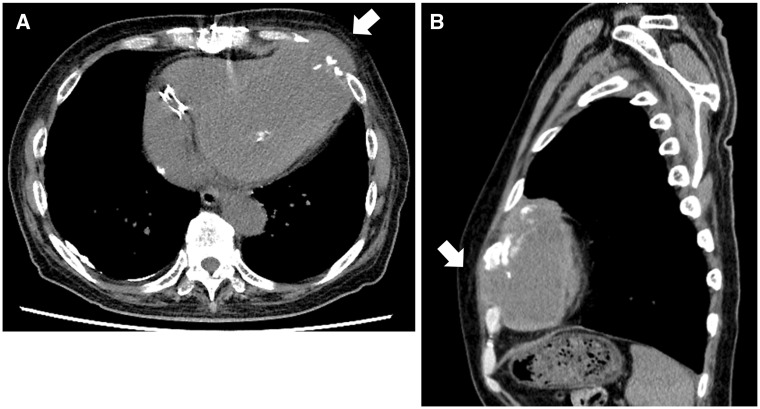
Computed tomography shows a pseudoaneurysm (white arrow) at the apex of the heart, which protruding outside of the thorax. (*A*) Transverse view. (*B*) Sagittal view.
